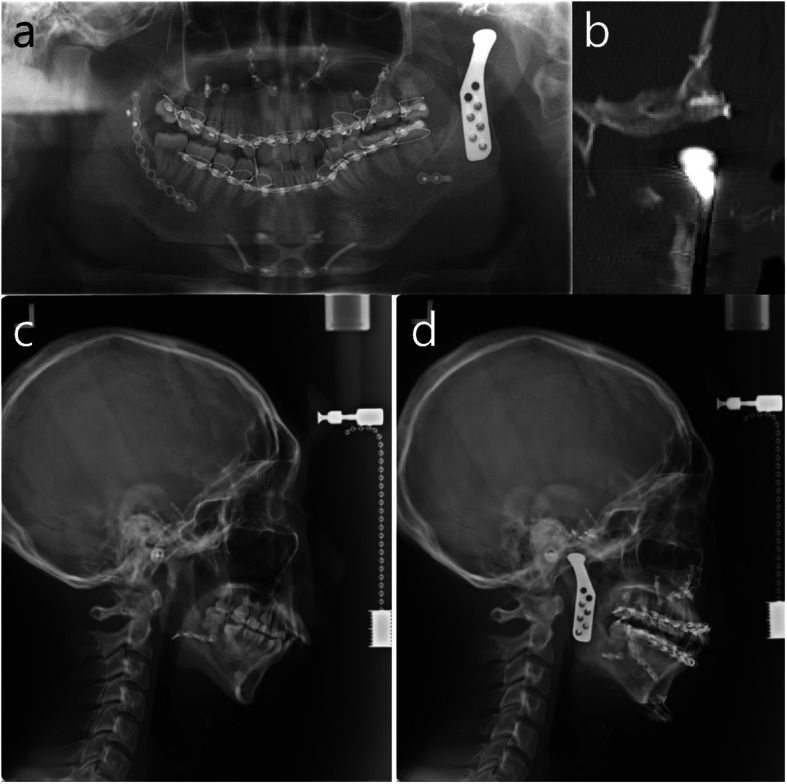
Radiographs after TJR and two jaw surgery in the third surgery. a panoramic view: TJR on the left side, b coronal cut of CT at TMJ. Installed fossa part of TJR on the well regenerated bone graft at temporal bone and mandibular part of TJR, c lateral cephalogram before two jaw surgery and TJR, d lateral cephalogram after two jaw surgery and TJR
